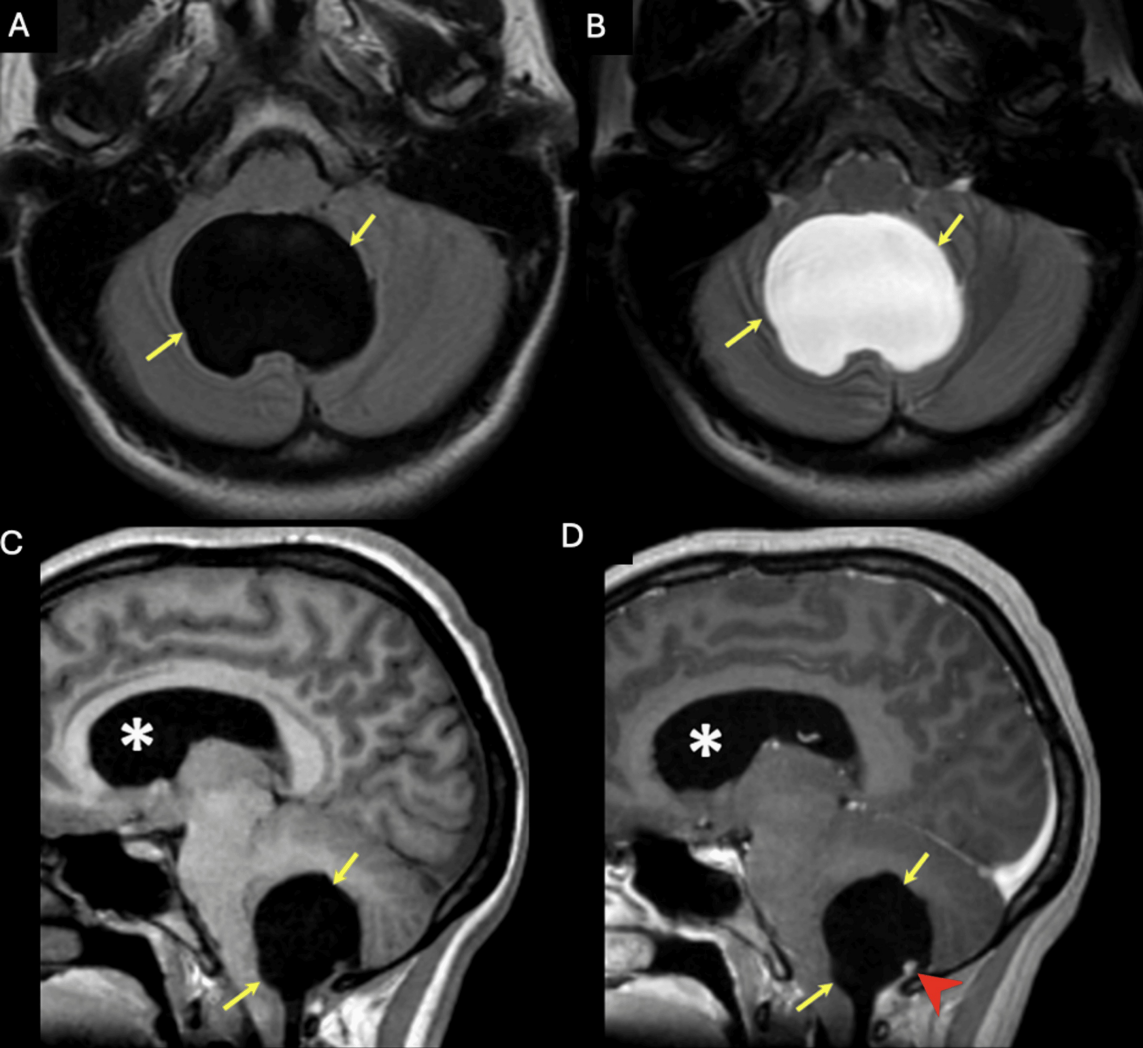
A) Axial FLAIR and B) axial T2WI MRI of the brain showed a cystic extra-axial lesion located at the inferior aspect of the cisterna magna (yellow arrows); C) Sagittal T1WI without contrast and D) sagittal T1WI postcontrast showed an enhancing nodule (red arrowhead) and dilation of the lateral ventricle (asterisk) secondary to obstructive hydrocephalus
